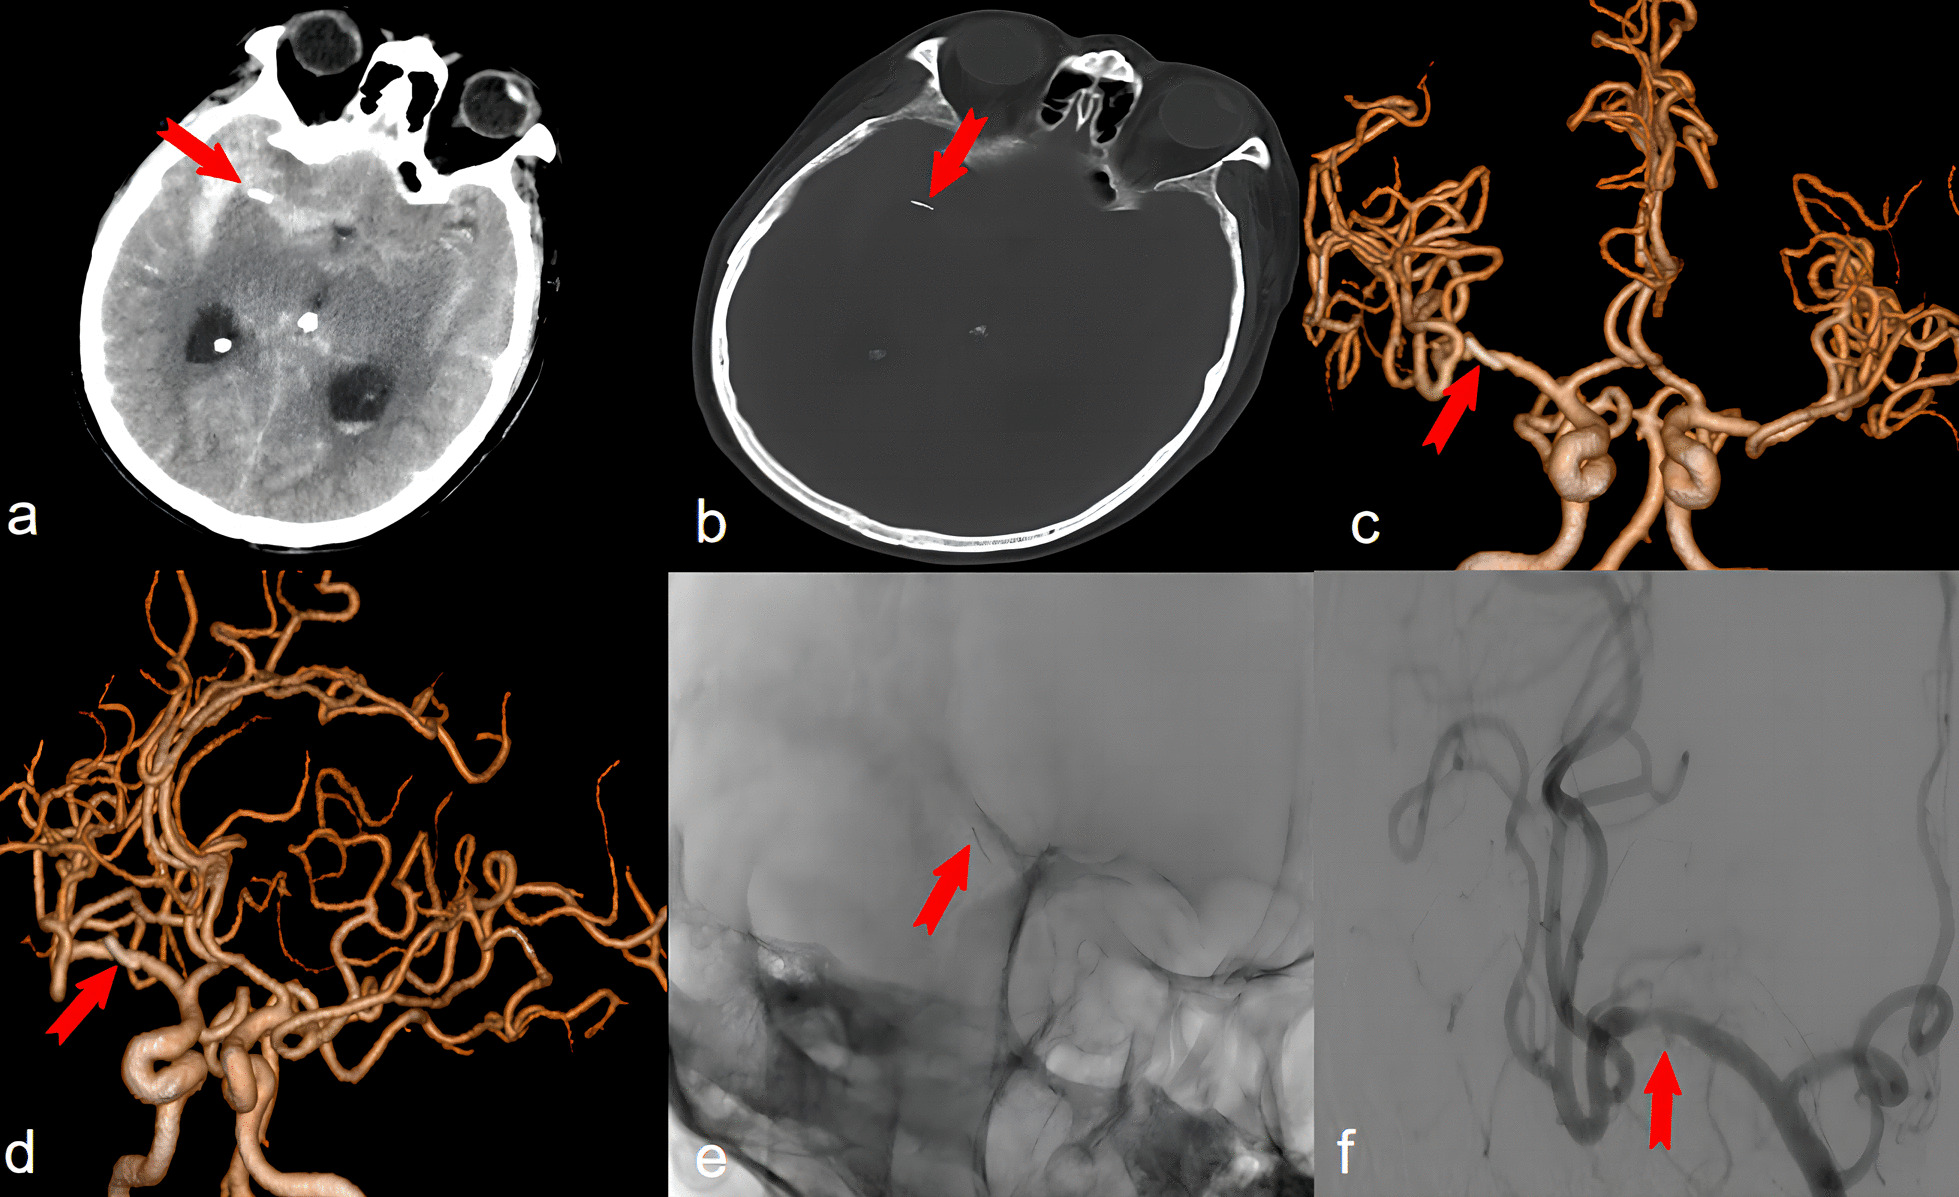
a Brain computed tomography scan showing diffuse subarachnoid hemorrhage; a short streak-like high-density shadow is seen next to the M1 segment of the right middle cerebral artery. b Bone computed tomography scan with evidence of a short strip-like dense shadow in the right cerebral hemisphere. c, d Computed tomography angiography (CTA) of the head and neck shows calcified plaques with severe luminal stenosis and foreign body presence at the M1 bifurcation of the right segment of the middle. e, f Cerebral angiogram showing a foreign body penetrating the middle cerebral artery with a saccular aneurysm arising from the inferior wall, which was considered a intracranial pseudoaneurysm. The red arrow in a–f indicates a foreign body
